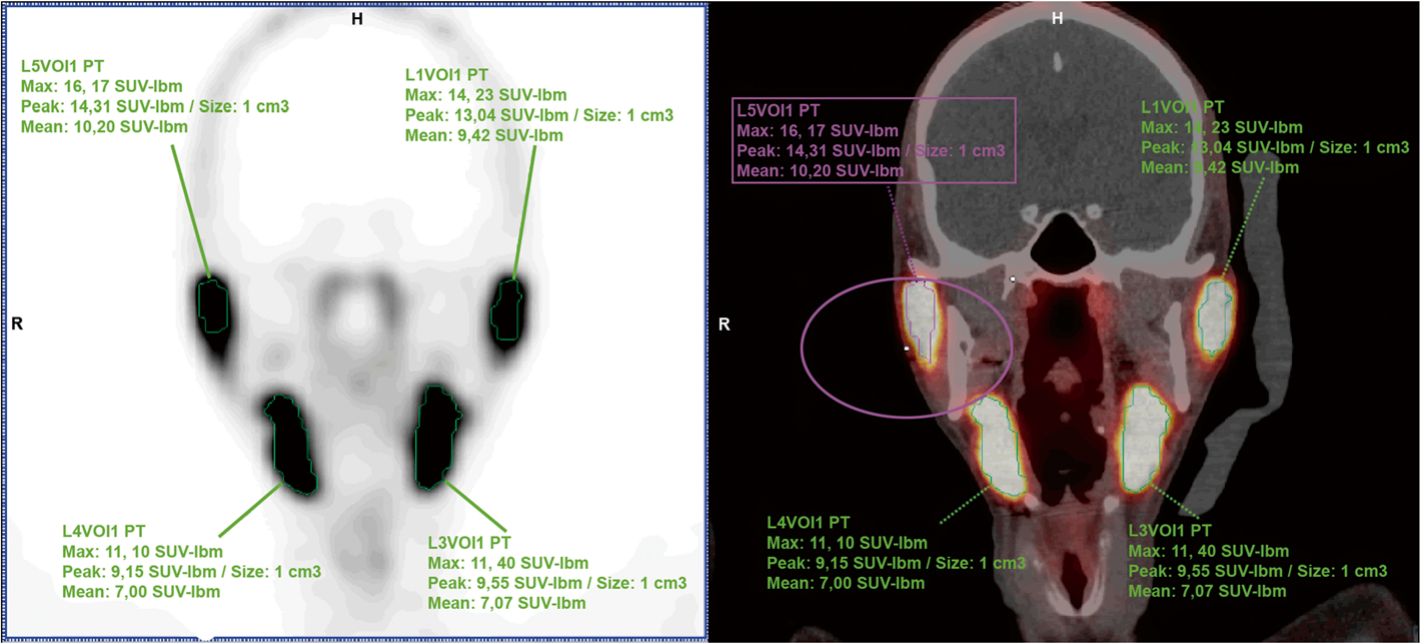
Measurements on PSMA uptake in both parotid glands in a patient who was scanned with a left-sided icepack. Differences in PSMA uptake were observed, when comparing the cooled (left) side to the non-cooled (right) side
